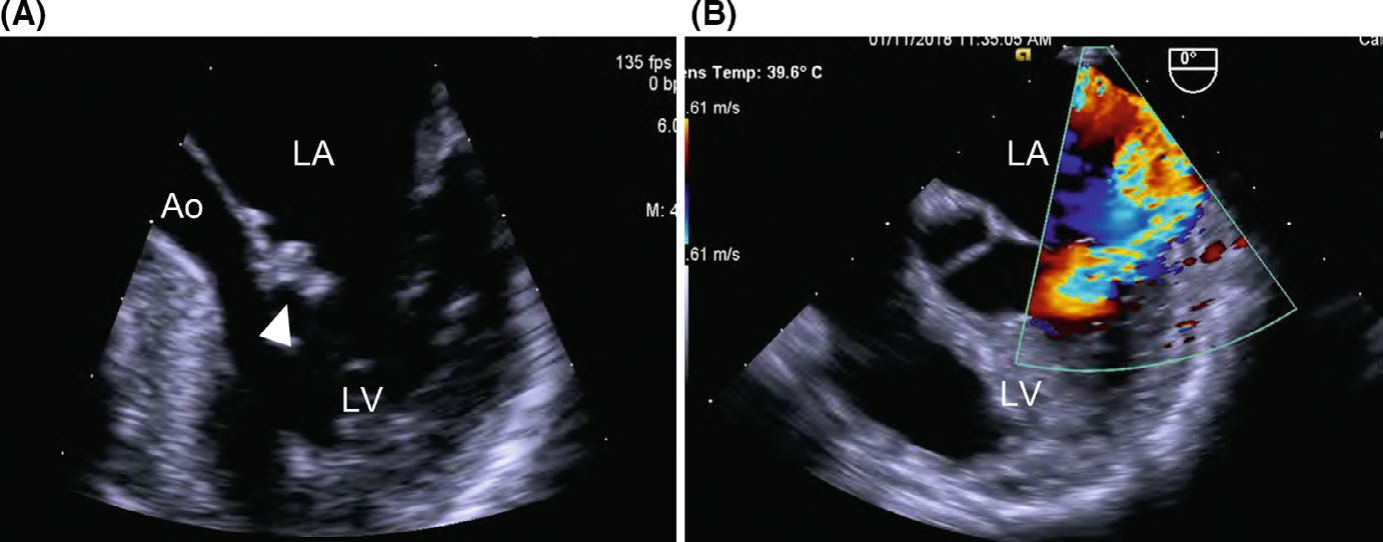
Transesophageal echocardiography on admission. There is a 5‐mm motile vegetation (arrow head) on the anterior cusp of the mitral valve (A) and mitral valve regurgitation (B)
